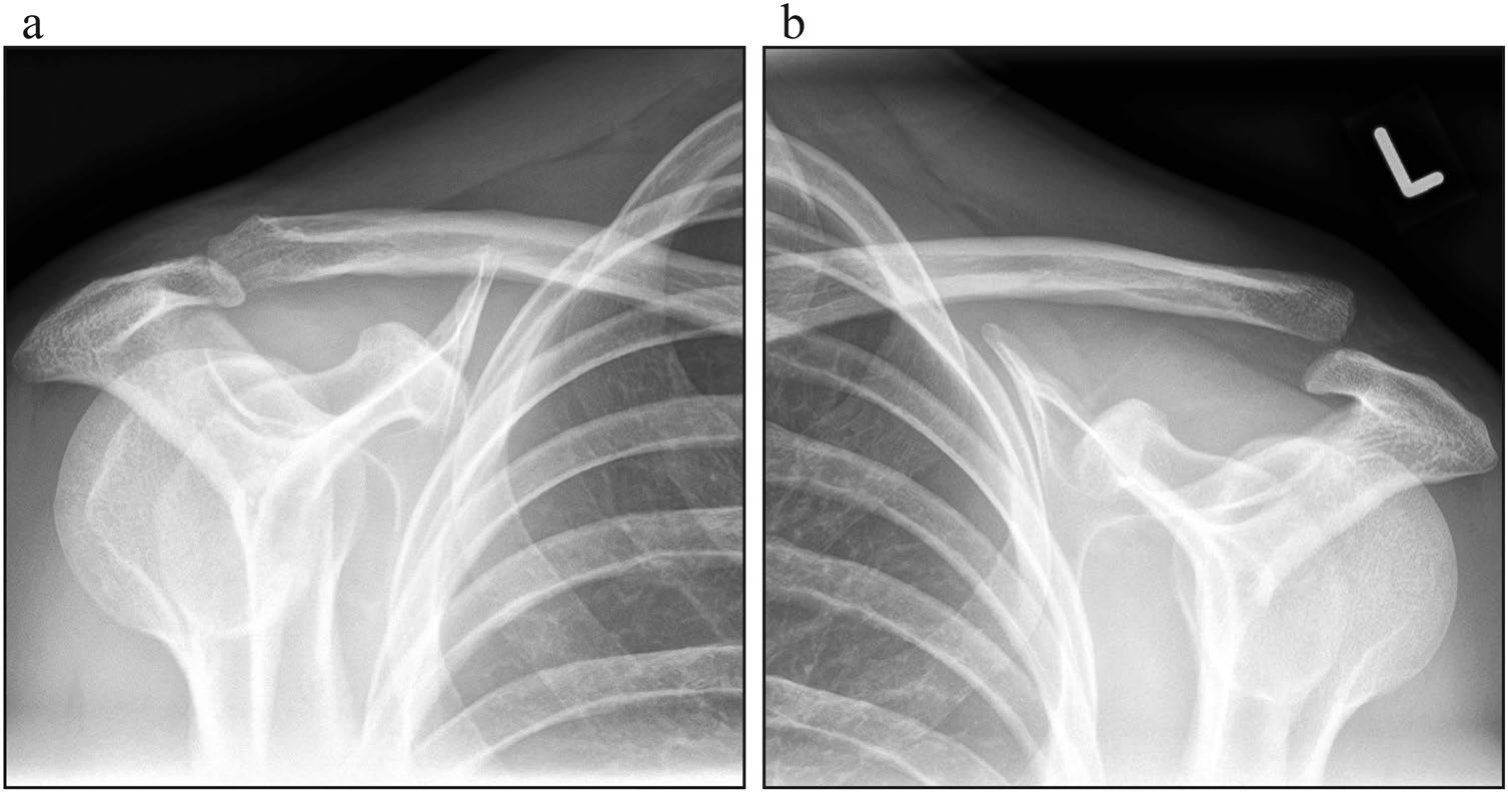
Bilateral Alexander X-ray views. a Unweighted bilateral AP axial oblique Alexander X-ray views obtained of the healthy right and b injured left AC joint on the day of injury
